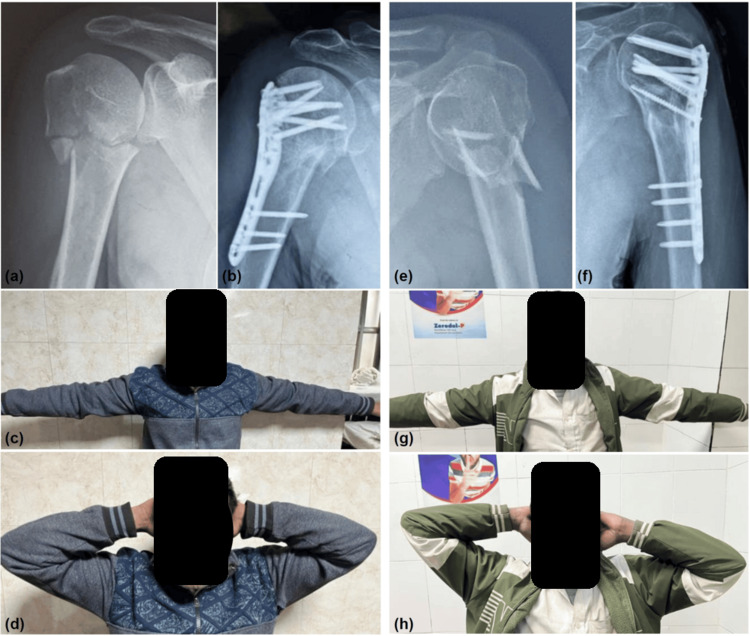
Preoperative radiographs and clinical photographs at the one-year follow-up visit a-b) Preoperative X-ray and X-ray at 1-year follow-up, respectively, of patient no. 15 of the study. c-d) Clinical photographs taken at the 12-month follow-up visit demonstrating the functional outcome of patient no. 15. e-f) Preoperative X-ray and X-ray at 1-year follow-up, respectively, of patient no. 13 of the study. g-h) Clinical photographs taken at the 12-month follow-up visit demonstrating the functional outcome of patient no. 13.
